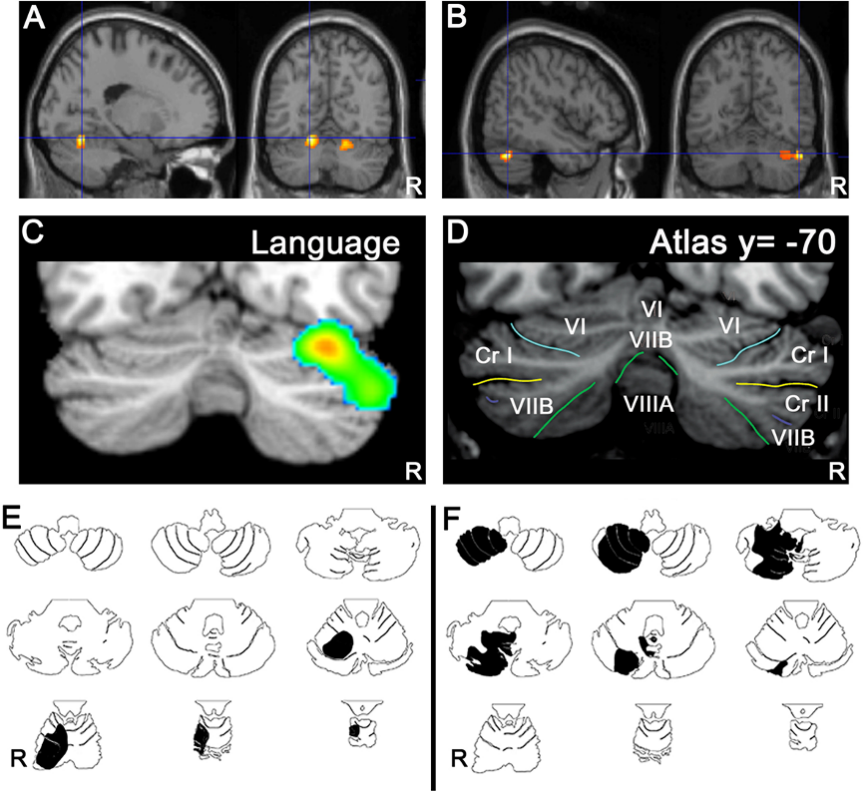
Topographic arrangement in cerebellum of speech versus language representation. Functional MRI localizes articulation (A) to medial parts of lobule VI bilaterally, whereas verb generation (B) activates lateral regions of lobule VI and Crus I on the right. In a meta-analysis of functional imaging studies [24] higher level language tasks engage the right lateral posterior cerebellum, lobules VI and Crus I (C) according to the lobule identification in (D; [23]). Case studies of cerebellar stroke patients reveal topography for articulation vs. higher-level language tasks. A patient with stroke in the territory of the right superior cerebellar artery (E, black shading) involving lobules I-VI was dysarthric; whereas a patient with stroke in the territory of the right posterior inferior cerebellar artery (F, black shading) involving lobules VII-IX was not dysarthric but performed poorly on the Boston Naming Test [25, 26] (from [2]).
